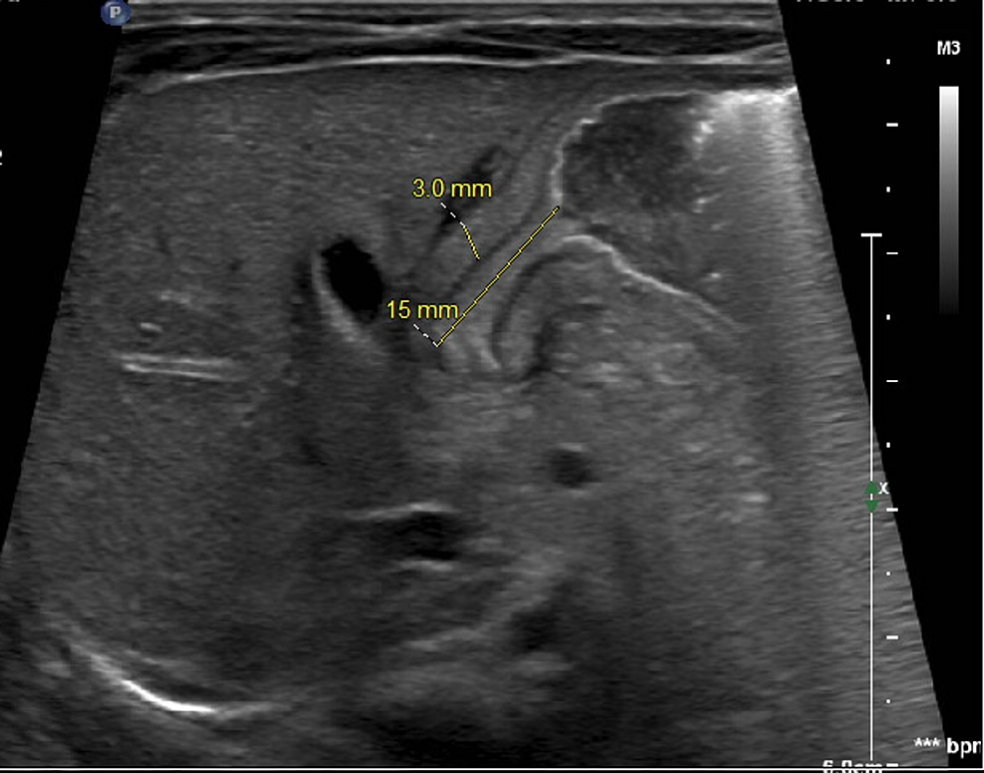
Transabdominal ultrasound demonstrating pyloric wall thickening of 3 mm and elongation of the pylorus 15 mm as represented by dotted yellow lines.
